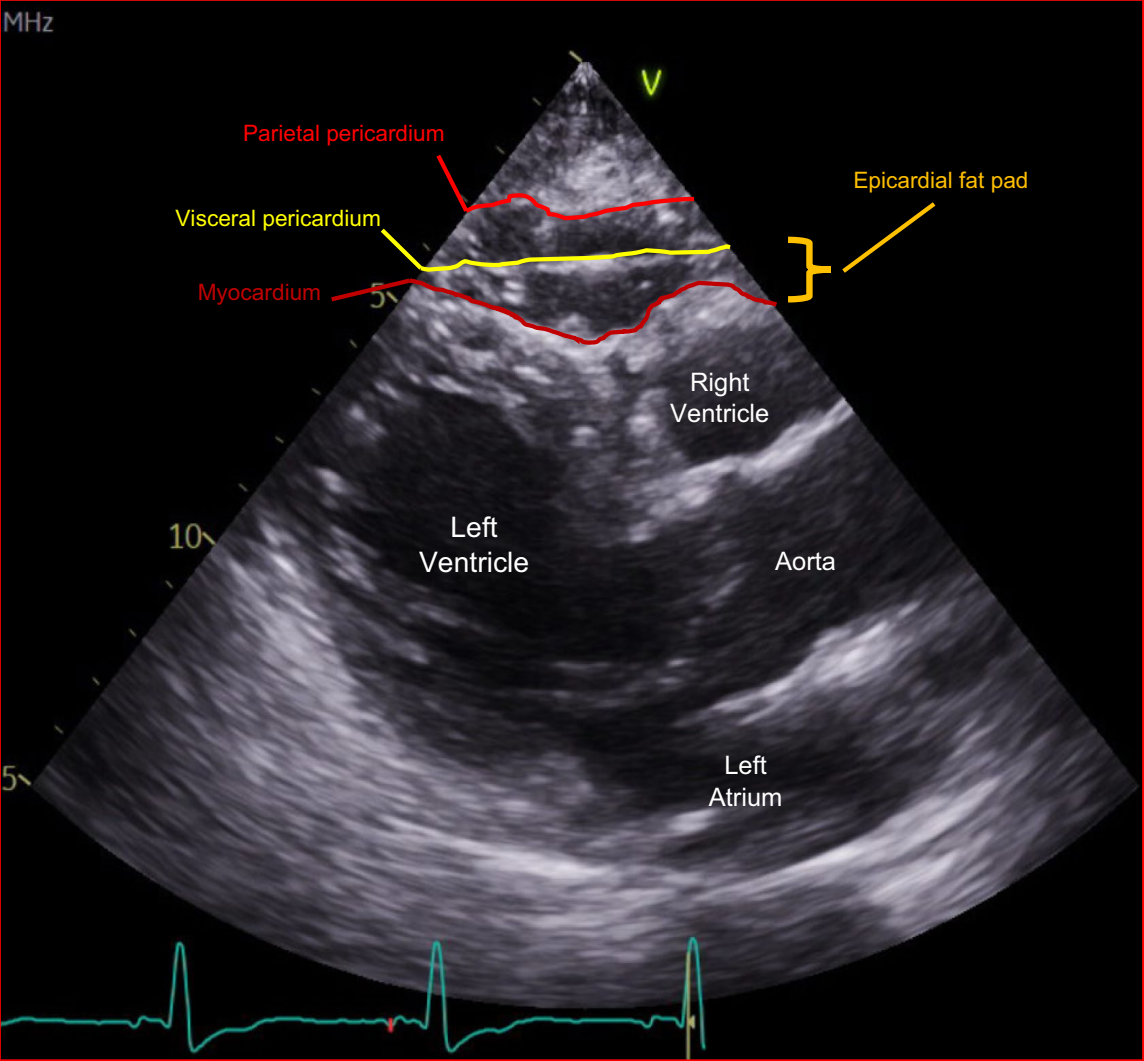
Parasternal long axis window from transthoracic echocardiogram, depicting a layer of epicardial fat between the myocardium and epicardium
